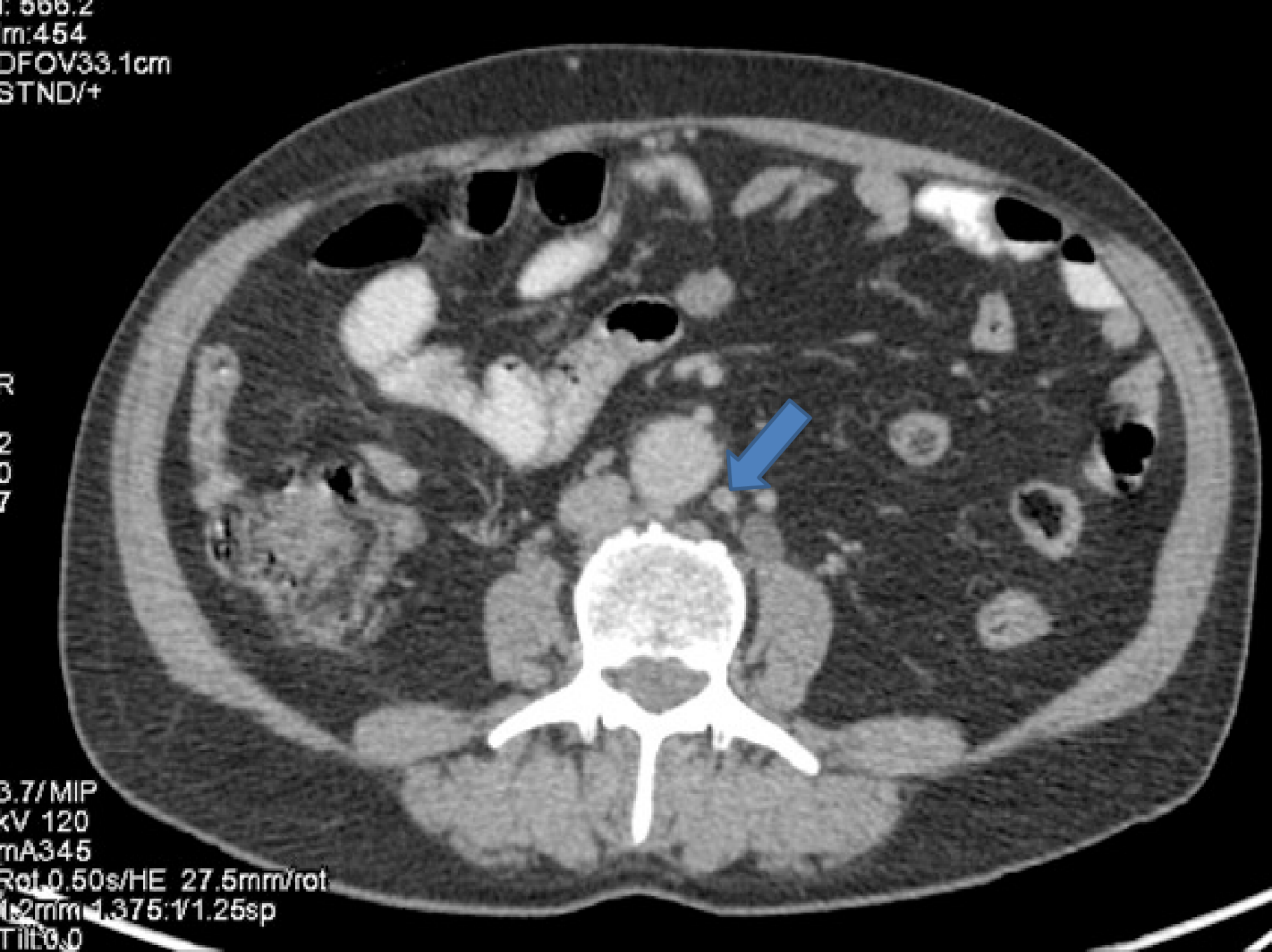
Computed tomography (CT) showing a para-aortic lymph node measuring about 1 cm from where fine needle aspiration cytology (FNAC) was positive for malignant cytology.
